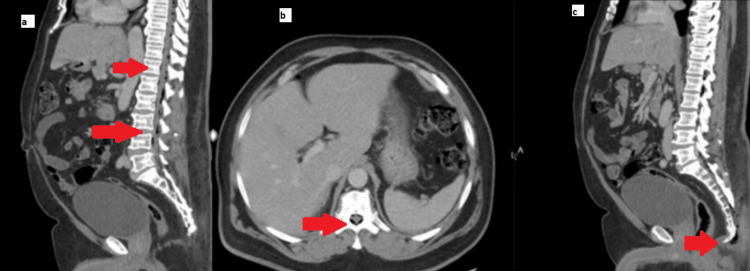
Computed tomography scan showing extensive soft tissue air extending into the spinal canal with multiple air foci in the epidural space of the thoracolumbar spine, concerning for sacrococcygeal infection/inflammation extending through the spinal canal, recommend Magnetic Resonance Imaging to exclude epidural intraspinal abscess. (A) Sagittal image, extensive soft tissue air extending into the spinal canal with multiple air foci in the epidural space of the thoracolumbar spine (B) Axial image, showing air within the spinal column (C) Sagittal image, subcutaneous tissue stranding with multiple air foci at the sacrococcygeal region
